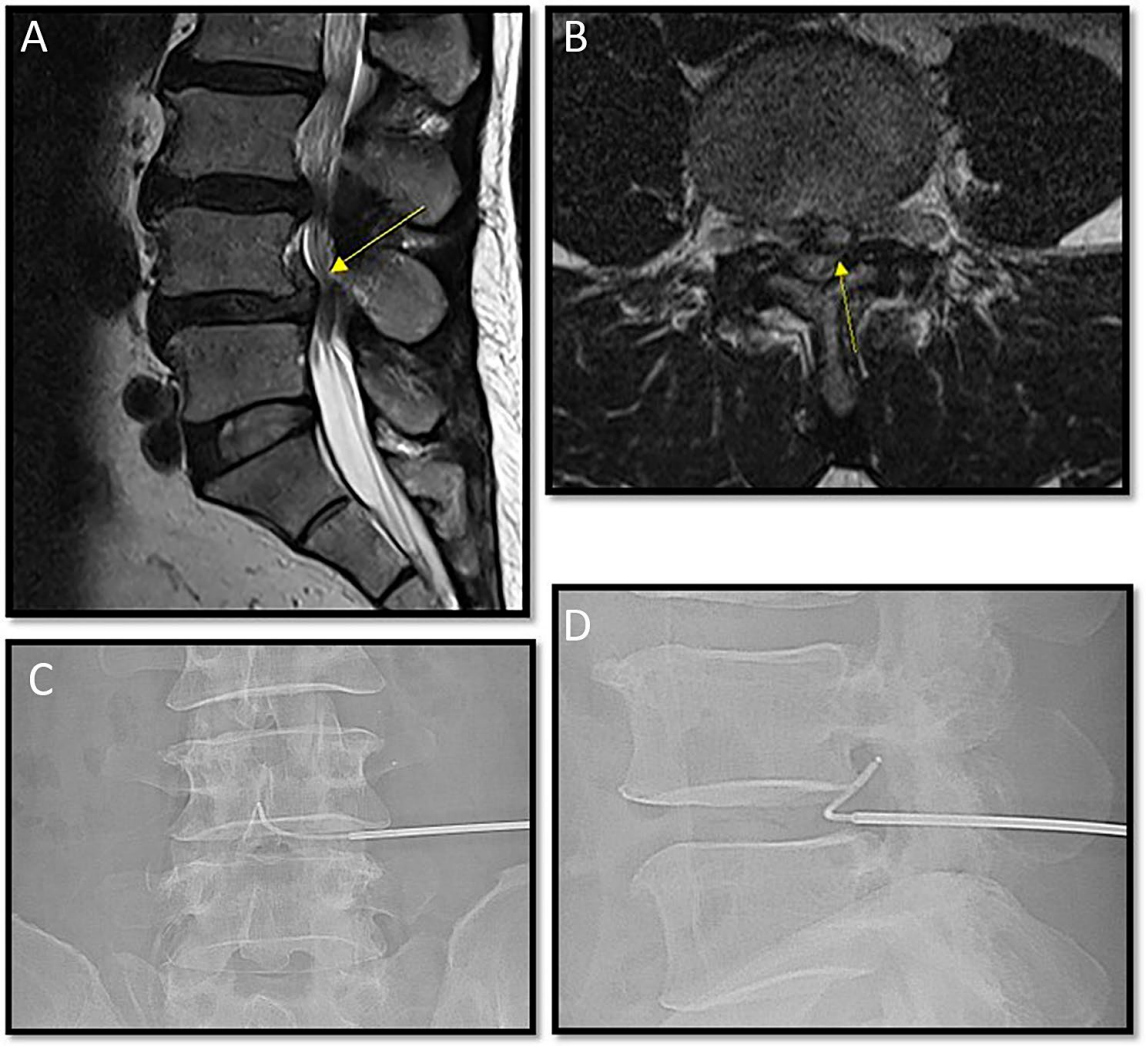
Magnetic resonance imaging (MRI) and intra-procedural fluoroscopy images of a 65-year-old man (case 1). (a, b) Sagittal and axial lumbar MRI T2-weighted images reveal spondylolisthesis of the fourth lumbar spine and uncontained lumbar disc herniation characterized by upward migration of the nucleus pulposus due to fibrous annulus rupture at the fourth to fifth lumbar intervertebral discs. The displaced nucleus pulposus is situated in the left paracentral direction, causing compression of the dural sac and the left fourth lumbar nerve root. (c, d) During percutaneous lumbar nucleoplasty performed with the patient under local anesthesia, fluoroscopy demonstrates successful access as the radiofrequency (RF) probe navigates through the torn opening of the fibrous annulus and follows the upwardly displaced disc fragment deep within the spinal canal. RF plasma was administered from this location to the interior of the disc for approximately 10 min
